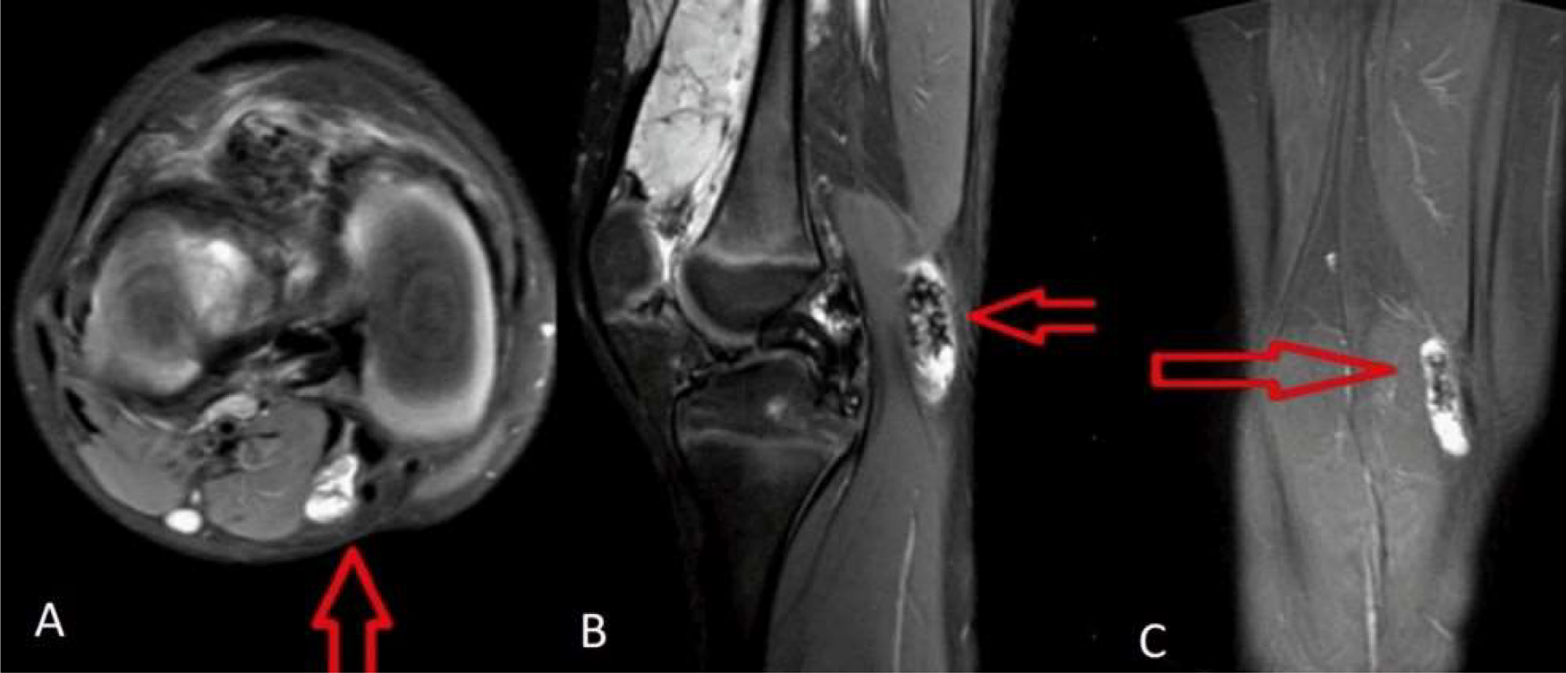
Magnetic Resonance (MRI) showing morrant bakers cyst in right popliteal fossa region (red arrow). A-MRI axial section, B-MRI sagittal section C-MRI coronal section.
